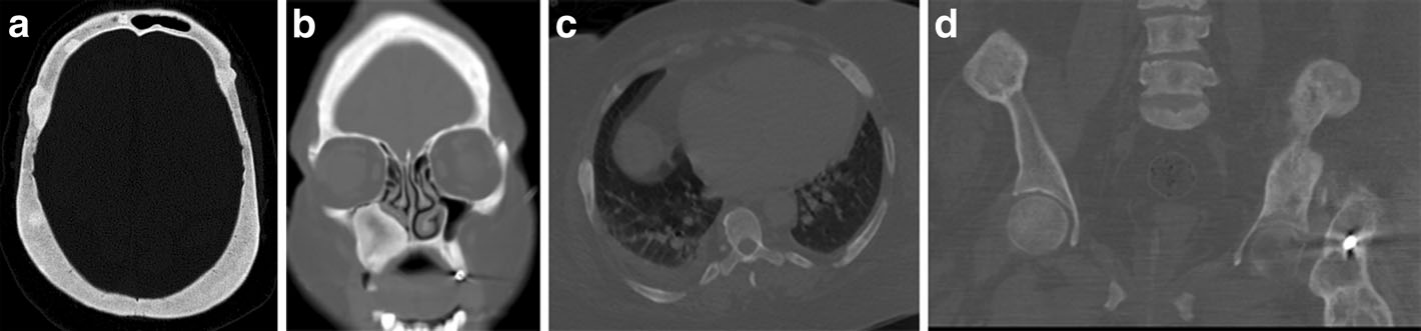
CT showed multiple expansile mixed lytic/sclerotic lesions in the calvarium, right maxillary antrum, ribs and iliac crests bilaterally
